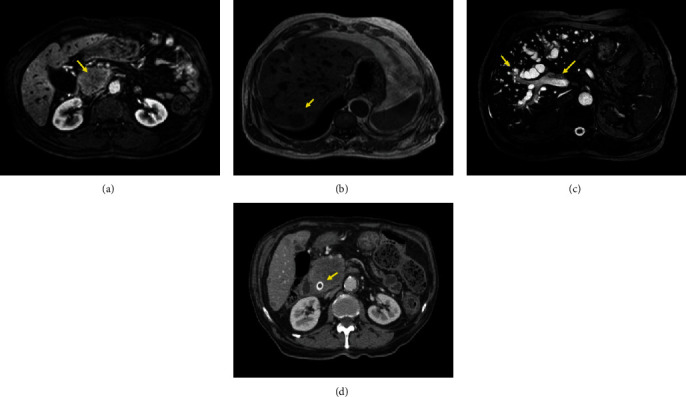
MR imaging of the pancreatic head mass (a) of the larger liver metastasis (b) and of the biliary dilatation (c). CT imaging of the biliary drainage (d).
